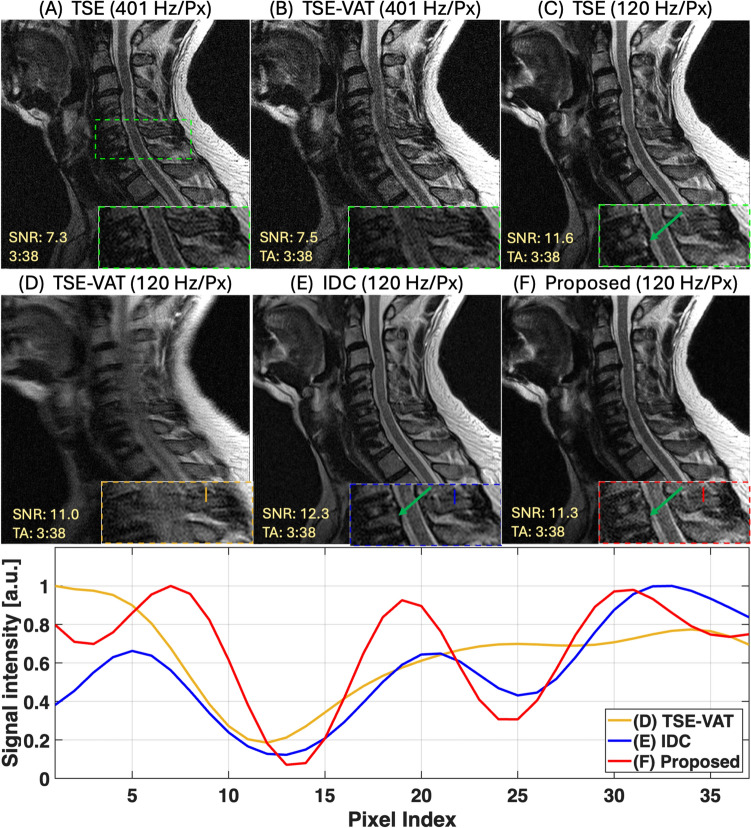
T2-weighted images of a 59-year-old male (BMI of 23.1 kg/m^2^) with anterior cervical discectomy and fusion (titanium). aSNR and acquisition time (TA) are labeled in the lower-left corner. Frequency-encoding direction is labeled by white arrows. A High-bandwidth TSE with less distortion but low aSNR; B TSE-VAT at 401 Hz/Px shows blurring and low aSNR; C low bandwidth (120 Hz/Px) TSE with higher aSNR but larger distortion and signal pile-up (green arrows); D low-bandwidth (120 Hz/Px) TSE-VAT shows severe blurring; E IDC and F the proposed method applied to low-bandwidth RE-TSE reduce distortion around the screws (green arrows) while maintaining high aSNR. Line profiles for the low-bandwidth case are shown to compare the degree of smoothing across methods
